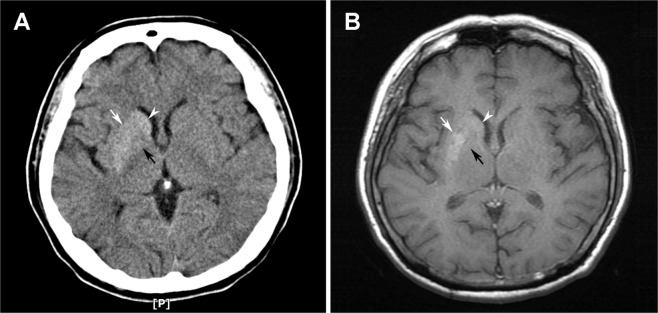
Typical images from (A) computed tomography (CT) and (B) T1-weighted magnetic resonance imaging (MRI) showing striatal anomalies of putamen (white arrows) and caudate nucleus (white arrowheads). Note the hyperdensity over globus pallidus on CT image but lack of hyperintensity on MRI (black arrows), defined as “incompatibility” in the present study.
